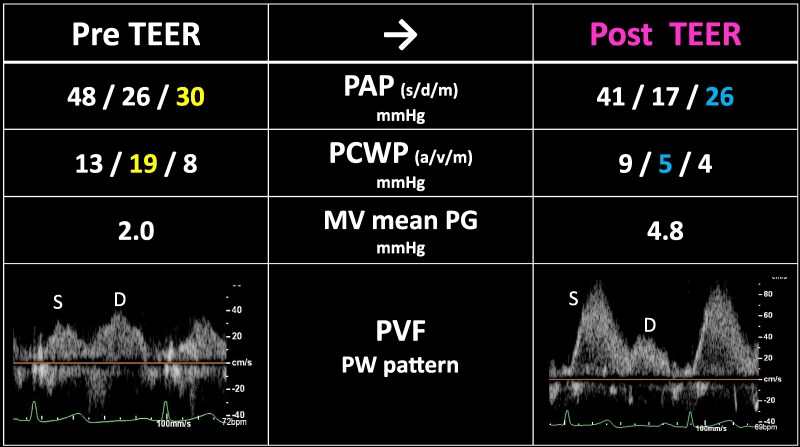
The changes of pressure parameters measured by right heart catheter and mean pressure gradient through mitral valve, and pulmonary vein flow pattern from transoesophageal echocardiography before and after transcatheter edge-to-edge repair (TEER). PAP, pulmonary artery pressure; PCWP, pulmonary capillary wedge pressure; MV, mitral valve; PG, pressure gradient; PVF, pulmonary vein flow; PW, pulse wave Doppler; S, systolic flow; D, diastolic flow.
